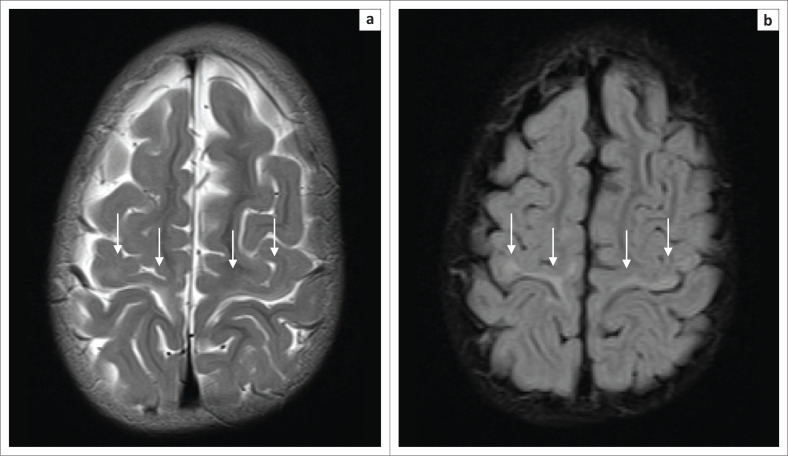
Three-year-old male child with history of neonatal encephalopathy, low Apgar scores and seizures. (a) An axial T2-weighted sequence image, which does not demonstrate the perirolandic changes adequately, and these changes would be difficult to diagnose without the axial FLAIR sequence image (b) which shows that the changes are almost exclusively involving the precentral gyrus.
